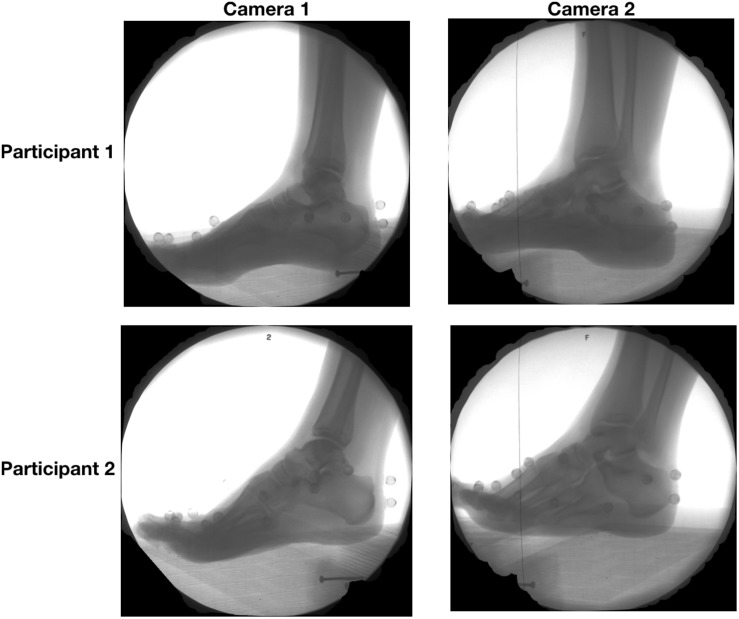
Radiographic images captured from camera 1 (left) and camera 2 (right) of two sample participants during the stance the phase of running.
